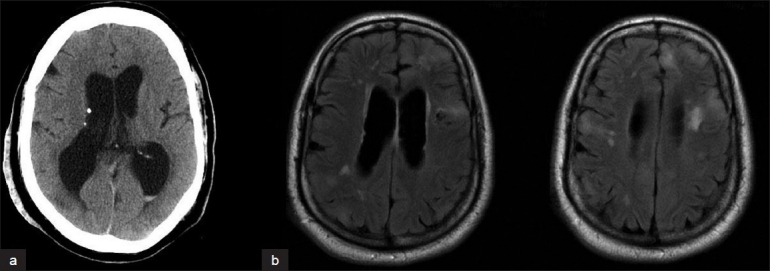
(a) CT brain showing periventricular calcifications and (b) MRI FLAIR sequence showing subcortical and periventricular white matter changes. Both of these findings are common in patients with a diagnosis of tuberous sclerosis
